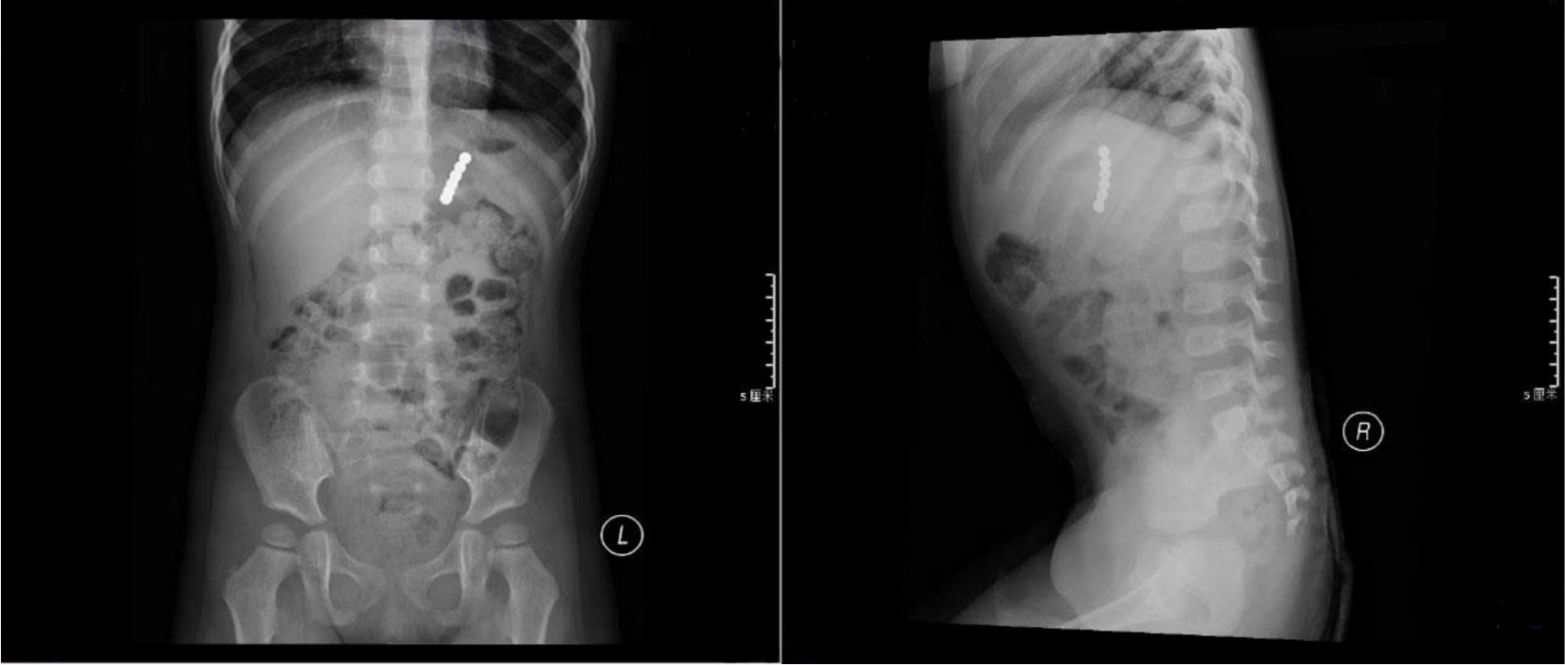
Anterior and lateral X-ray views of a patient who ingested a small number of magnetic beads.
